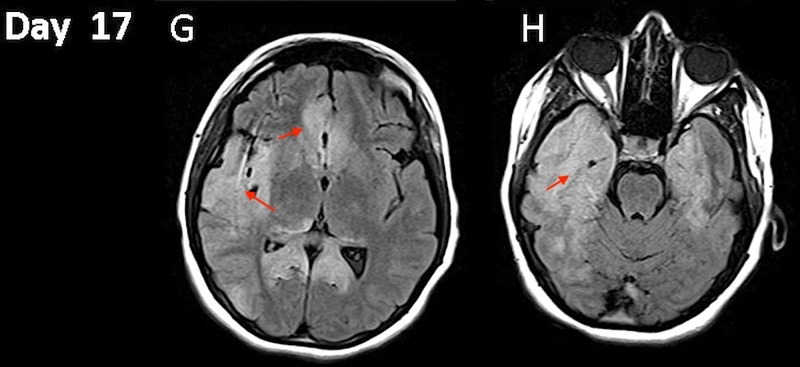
Magnetic resonance imaging (MRI). Hospital day 17 fluid attenuated inversion recovery (FLAIR) changes evolution (G and H).
